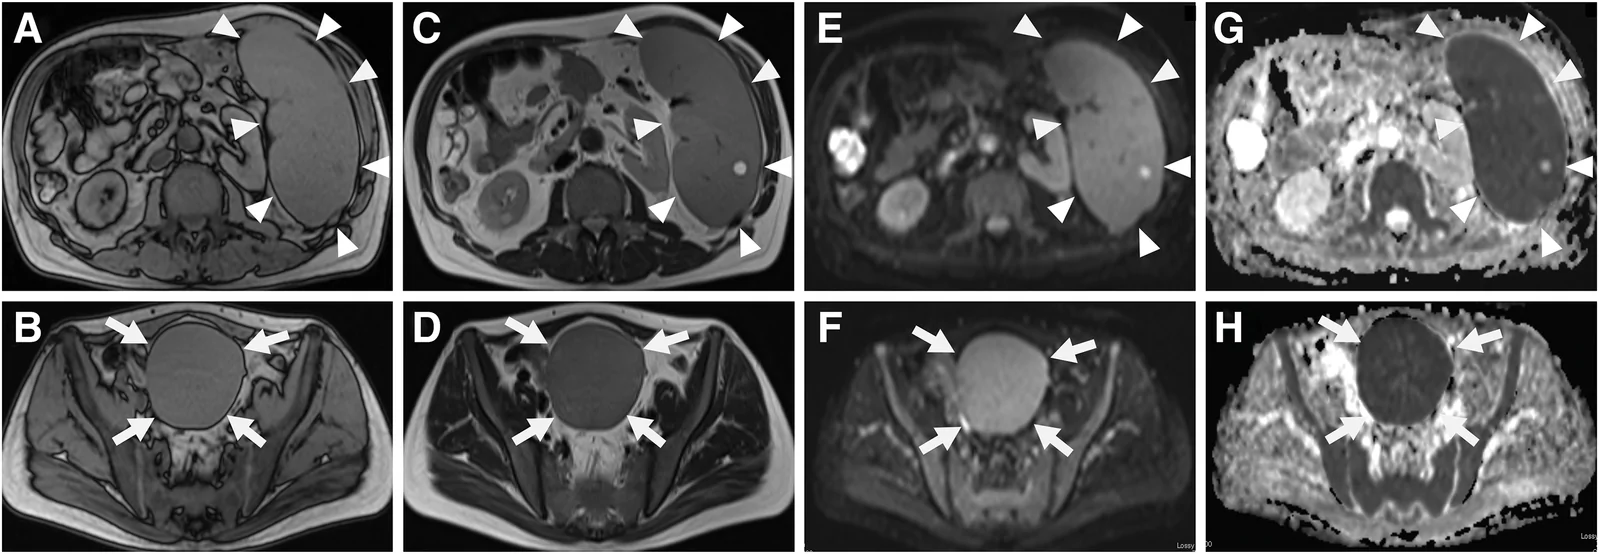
MRI findings at X–2 months. Each panel showed the spleen (upper row) and the pelvic mass (lower row), which demonstrated identical signal characteristics across all sequences (isointense on T1-weighted images, slightly hypointense on T2-weighted images, hyperintense on DWI, and low ADC values). (A) Axial T1-weighted image of the spleen (arrowheads). (B) Axial T1-weighted image of the pelvic mass (arrows). (C) Axial T2-weighted image of the spleen (arrowheads). (D) Axial T2-weighted image of the pelvic mass (arrows). (E) Axial DWI of the spleen (arrowheads). (F) Axial DWI of the pelvic mass (arrows). (G) Axial ADC map of the spleen (arrowheads). (H) Axial ADC map of the pelvic mass (arrows). ADC, apparent diffusion coefficient; DWI, diffusion-weighted imaging
